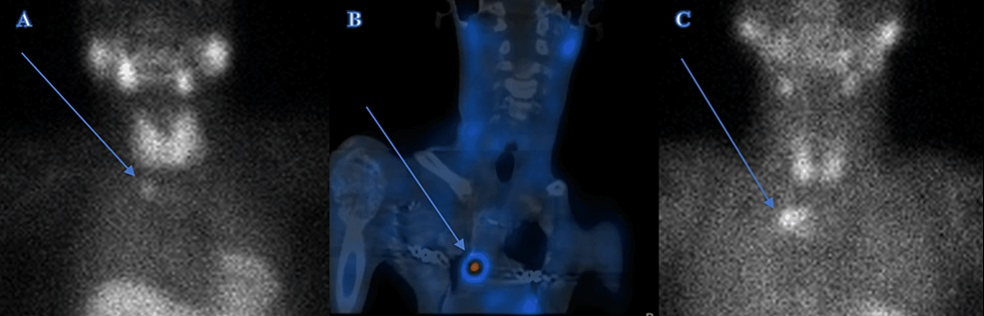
Tc99m-MIBI (methoxyisobutylisonitrile) scintigraphy of Case 1 (A), Case 3 (C), and single-photon emission computed tomography imaging (SPECT) scan of Case 2 (B) exhibited the mediastinal parathyroid adenomas (arrow).
